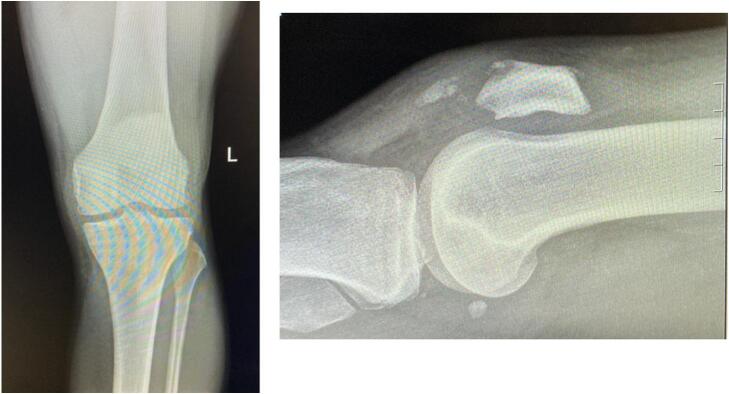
AP and lateral radiograph of left knee.
